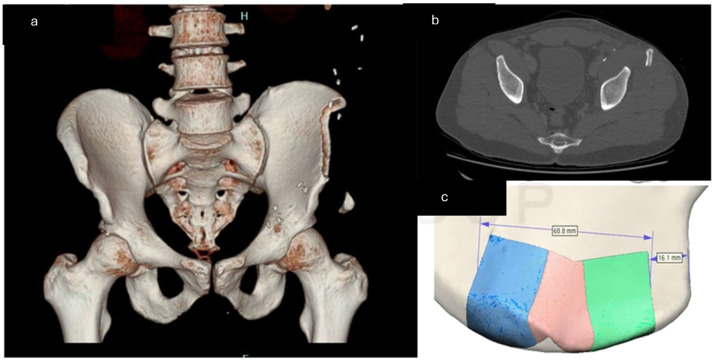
A Postoperative CT scan with bony reconstruction showing type A fracture. Fig. 1B. Avulsion fracture on axial cuts Fig. 1C. Virtual surgical planning demonstrated that 68.8 mm of the iliac bone crest was harvested with only 16.1 mm distance between the ASIS and the anterior osteotomy margin. The fracture was managed conservatively with activity modification and physiotherapy, resulting in satisfactory recovery.
